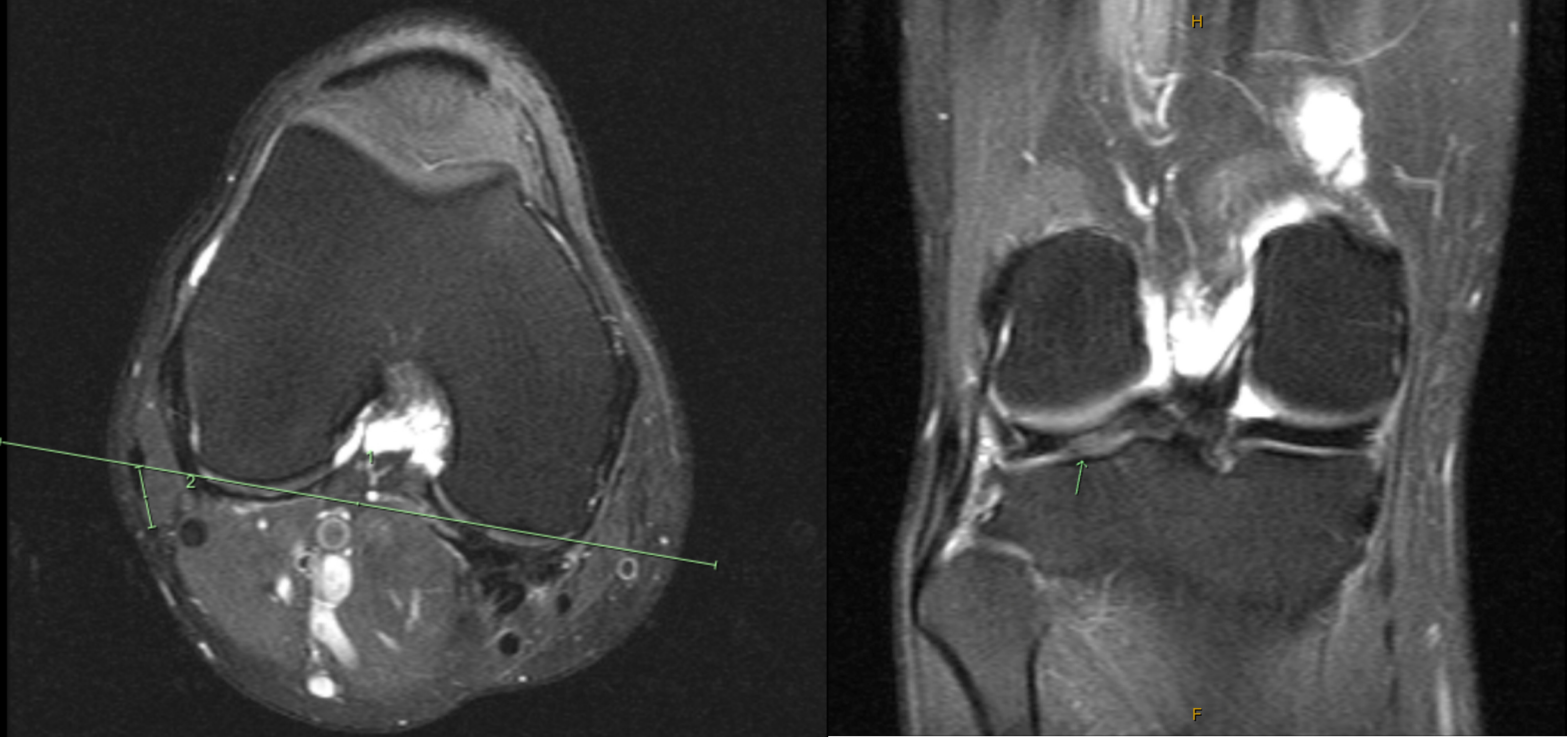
MRI of the right knee indicating no evidence of tear or peritendinous edema adjacent to the distal biceps femoris tendon or evidence of variant anatomy.
